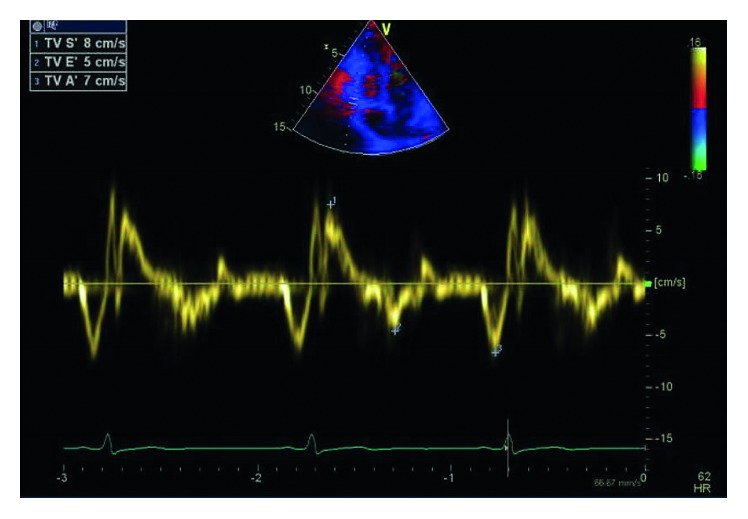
Tricuspid annulus tissue Doppler S wave of 0.08 m/s.
